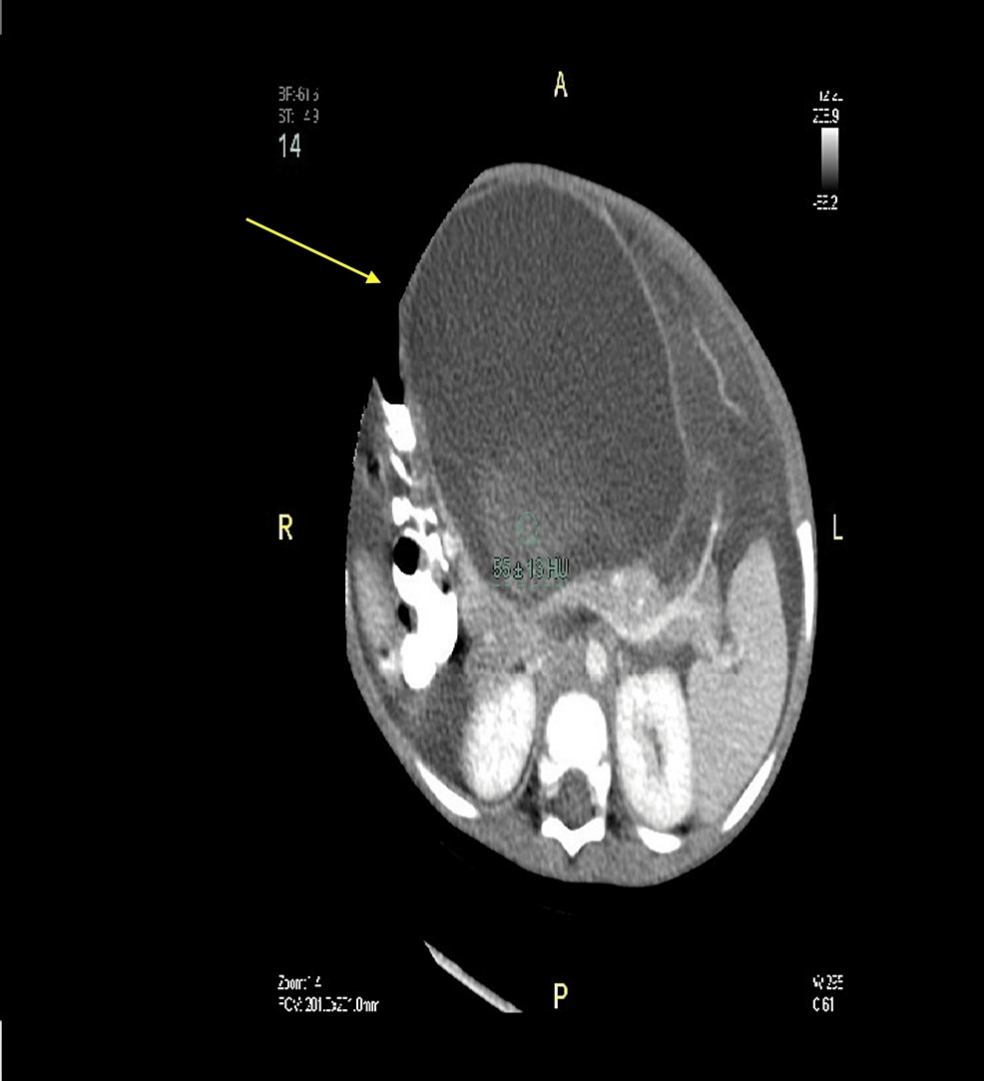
Abdominal CT scan showing pseudocyst in the head of the pancreas surrounded by fluid after follow-up (arrow) CT: computed tomography
